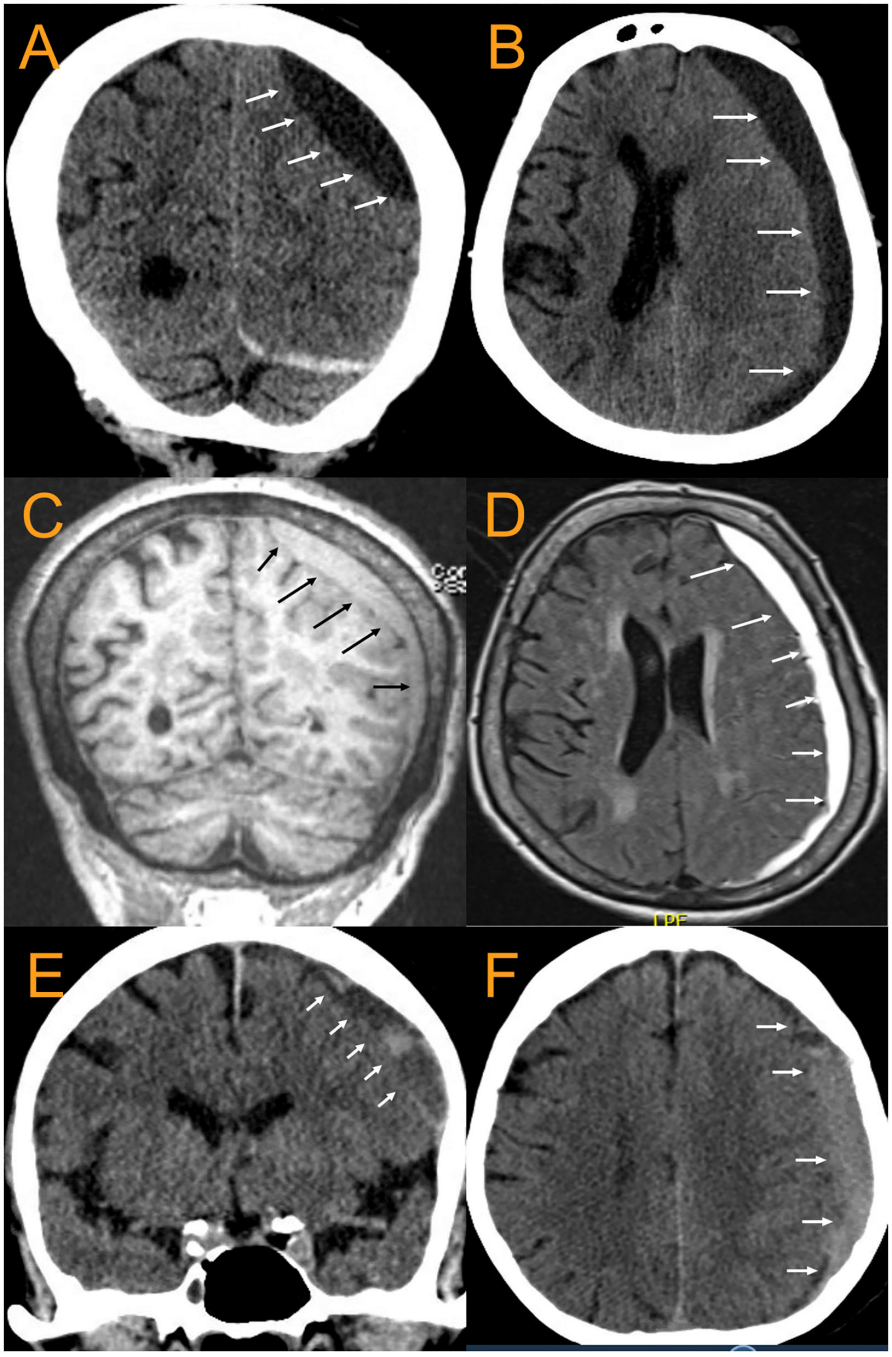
CT and MRI examples of cSDH. 88 yo F with largely chronic SDH with small acute component. (A). coronal CT, (B). Axial CT, (C). MPR coronal MRI, (D). T2-FLAIR Axial MRI. The arrows point to the inner subdural membrane which can be seen delineating a boundary between the cSDH and the pia -arachnoid. (E,F): 79 yoM with subacute cSDH. Once again, the arrows point out the location of the inner subdural membrane.
